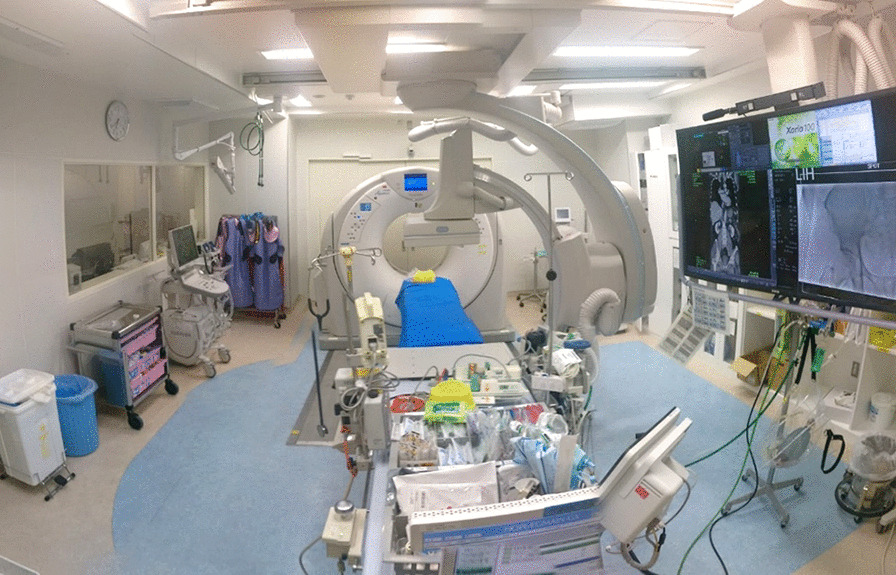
Photograph showing our IVR-CT system in the Hybrid ER (Aquilion PRIME, TSX-303B; Toshiba Medical Systems Corp., Tochigi, Japan). All life-saving procedures including airway management, emergency surgery, and transarterial embolization can be performed on the table without relocating the patient. a Sliding CT scanner with the two rows of rails on the floor, b CT examination and intervention table, c moveable C-arm, d 56-inch monitor screen, e ultrasonography equipment, and f mechanical ventilator. Unlike the usual CT scanner, after the patient is positioned on the CT examination and intervention table, the CT scanner moves to the table on rails with the table fixed. We use the room next to the Hybrid ER for surgical instrument storage and bring only what we need for the surgery into the Hybrid ER. The ventilator is located on the foot side of the trauma table. The blood refrigerator is located in the intensive care unit near the Hybrid ER. When an anaesthesiologist performs inhalation anaesthesia, the anaesthesia machine is located near the head side of the trauma table. Because the sliding CT scanner is moved to the adjacent CT suite after the CT scan, adequate space is available near the head side of the trauma table
